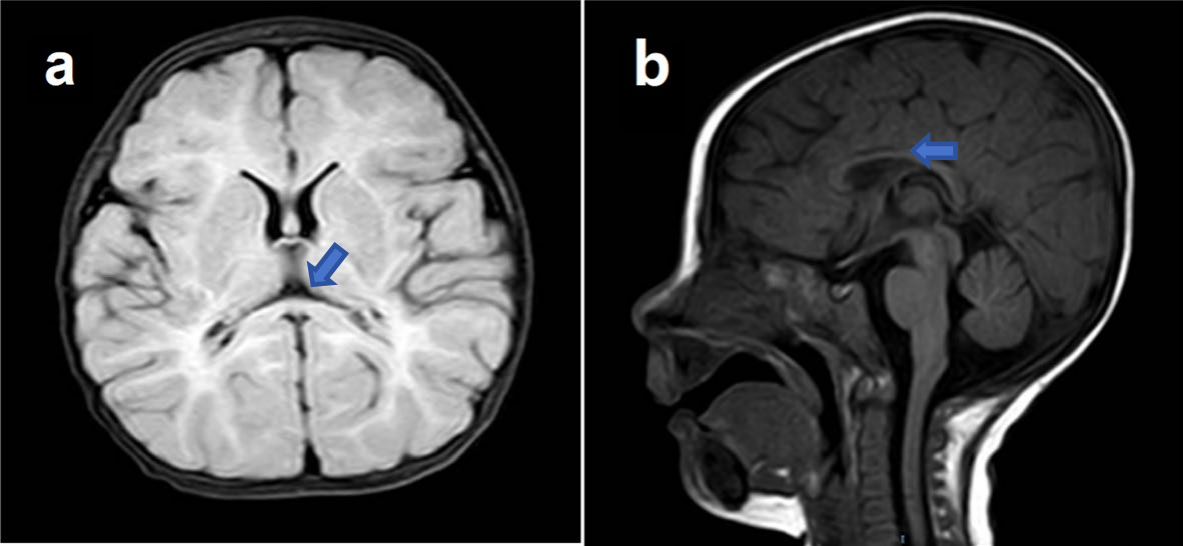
MRI showed weak white matter myelination signals of the corpus callosum (a, b)
